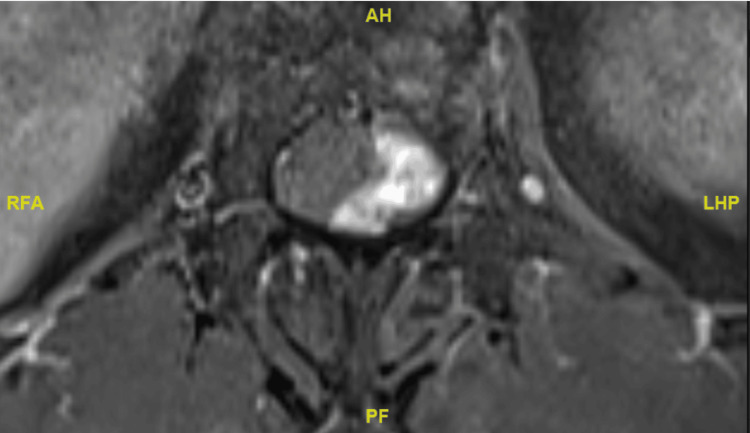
Pre-operative T1-weighted axial MRI image of intradural lesion at the level of T12.
